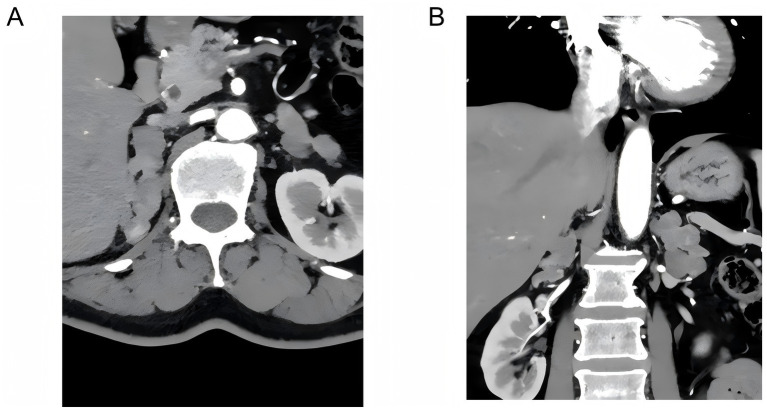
Preoperative computed tomography (CT) images of the abdomen demonstrating bilateral adrenal nodules. (A) Axial view. (B) Coronal view.
